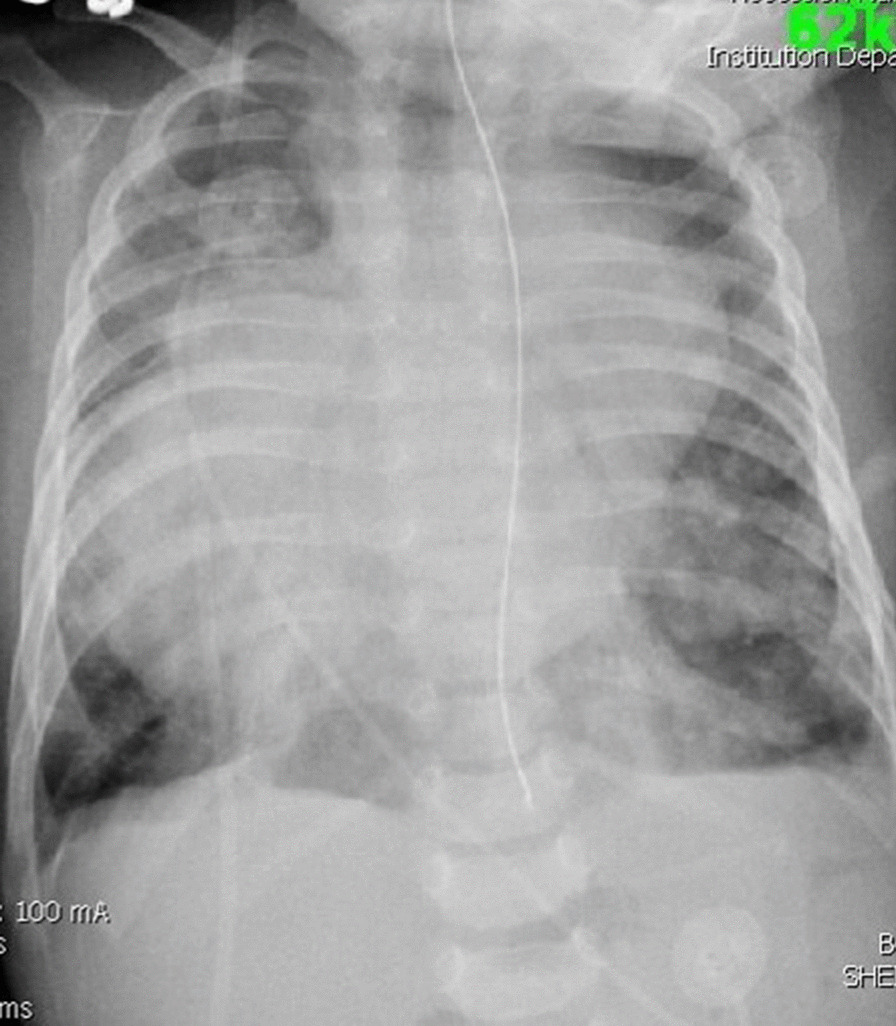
Plain chest radiograph showing features of cardiomegaly, dextrocardia and lung congestion
